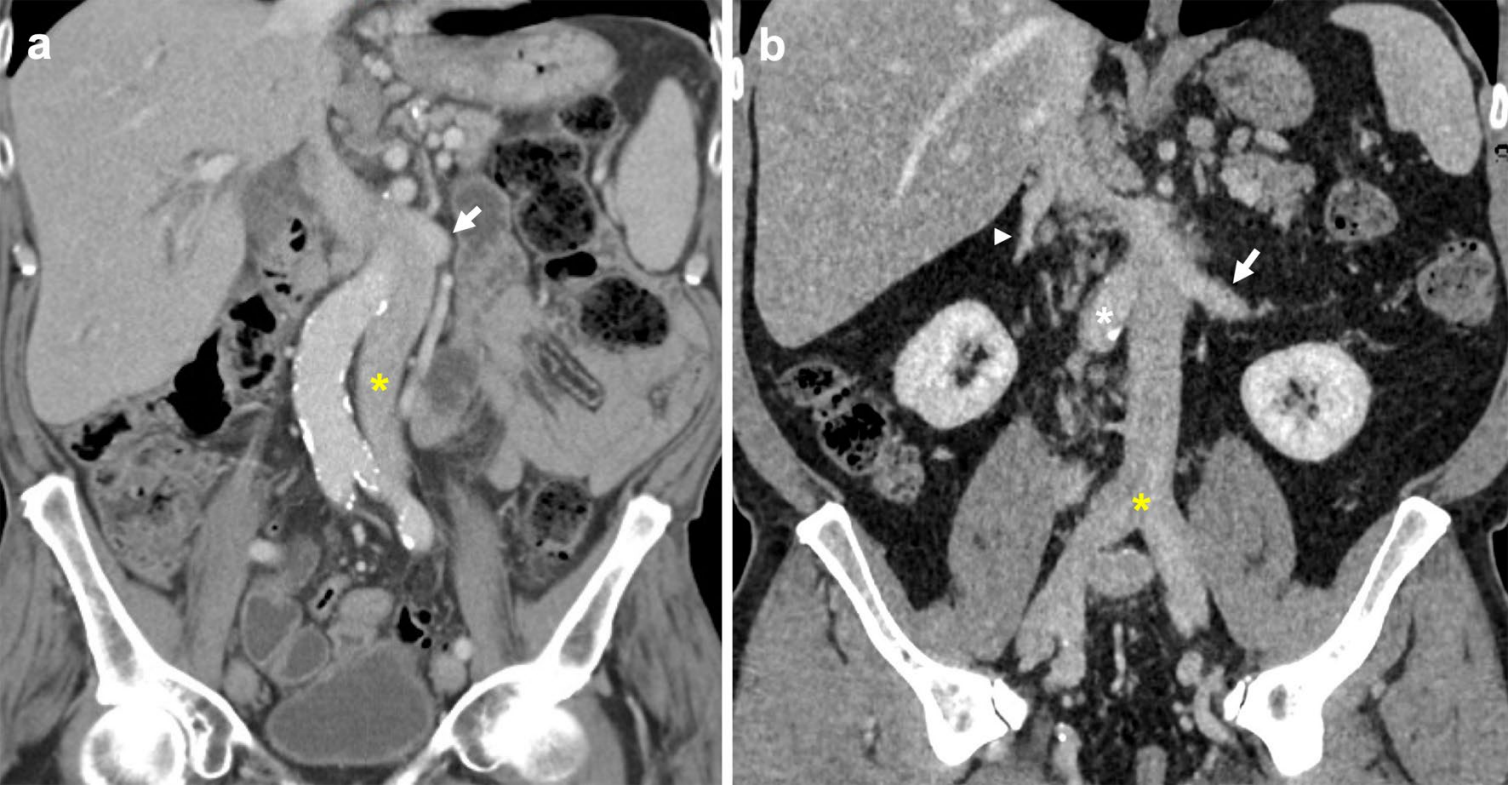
Left-sided IVC. a Coronal CT image shows a non-enhanced left-sided IVC (asterisk) draining into an enhanced left renal vein which connects to the hepatic IVC. b Coronal CT image of a second case demonstrates a typical confluence of common iliac veins (yellow asterisk) forming the infrarenal IVC on the left side of the aorta (white asterisk) to join the left renal vein (arrow) which drains into the orthotopic IVC. The proximal right renal vein (arrowhead) is also pictured draining into the suprarenal IVC
